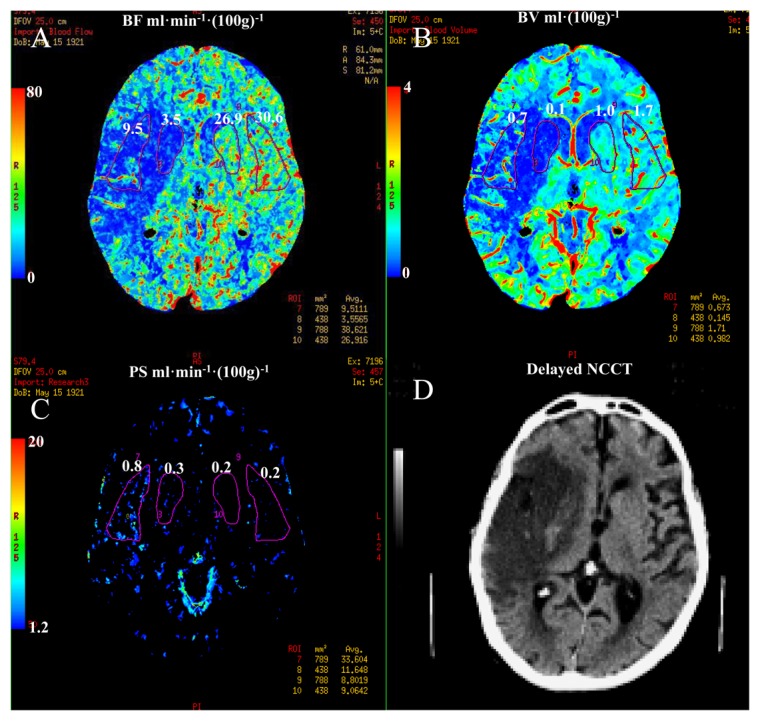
Permeability surface (PS) area product map from a patient with a large area of contrast leakage into the ischemic region: (A) CBF map of a 5 mm thick brain slice from the admission CTP study displayed with a color scale from 0 (dark blue) to 150 (red) mL·min^−1^·(100 g)^−1^ (B) of pixels with CBF less than 25 mL·min^−1^·100 g)^−1^; (C) corresponding PS map to CBF map in (a) showing the superimposed ischemic ROI and the mirrored ROI in the contralateral hemisphere. PS values in the ischemic and mirrored ROI were 0.40 and 0.122 mL·min^−1^·(100 g)^−1^ respectively; (D) corresponding delayed non-contrast CT scan of the same slice showing infarct and hemorrhagic transformation (red arrow). Courtesy of Chris d'Esterre, Richard Aviv and Ting-Yim Lee (University of Western Ontario).
